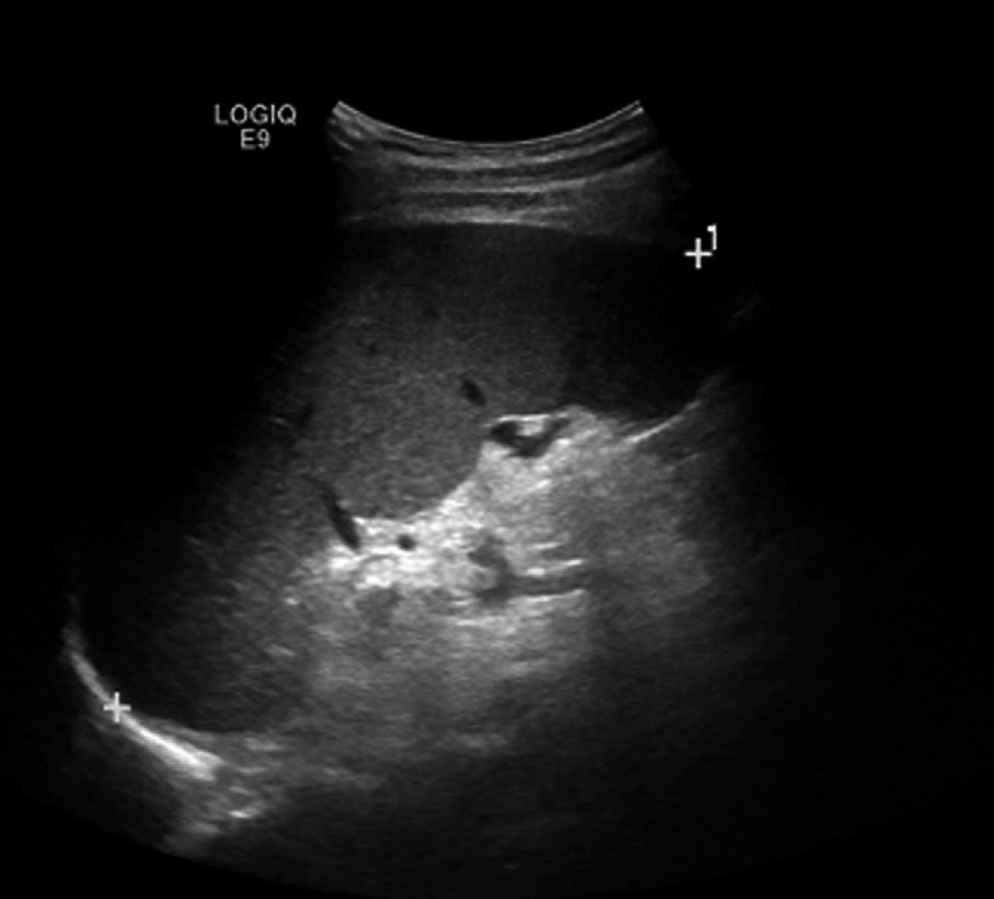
Ultrasound abdomen of Patient 2 showing massive splenomegaly
